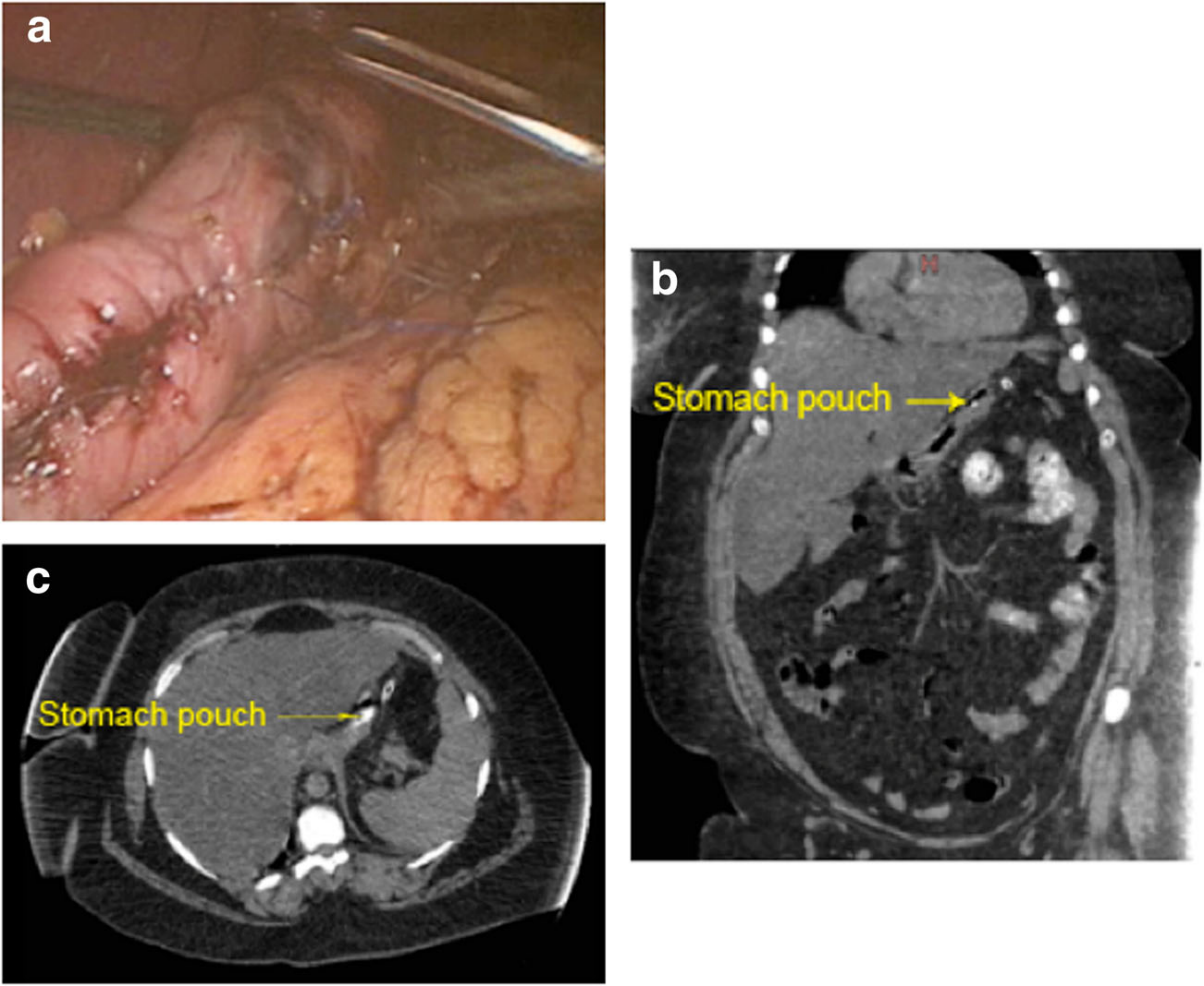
a Global view at the end of the procedure. Post-operative CT scan with oral contrast (b axial and c coronal views)
